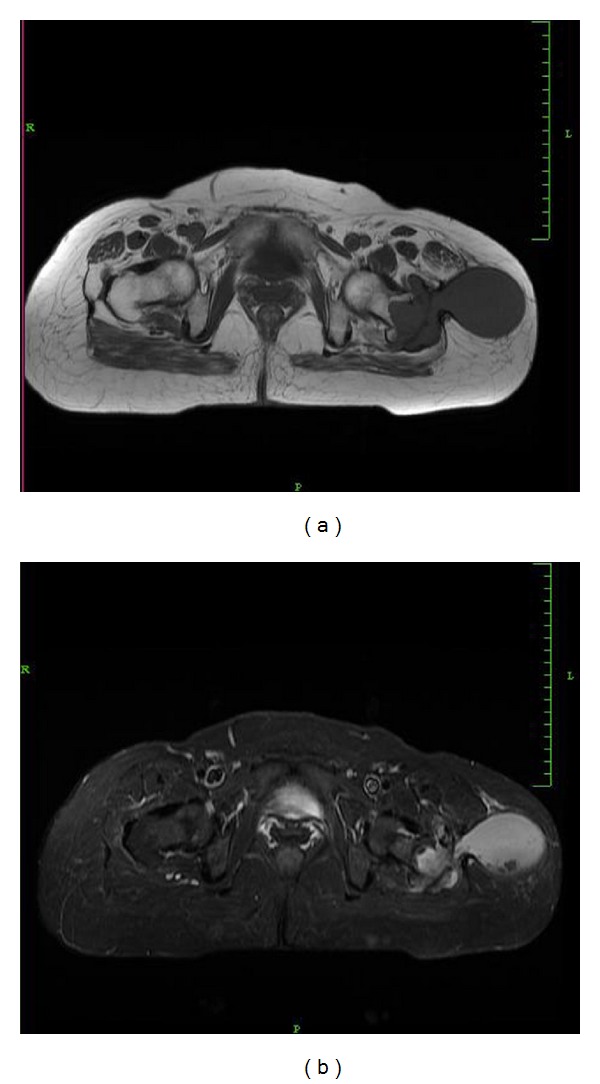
Axial T1- and T2-weighted MRI image of the pelvis showing the abscess extending from the left greater trochanter into the subcutaneous tissues laterally through a small defect in the deep fascia resembling a dumb-bell-shaped abscess.
